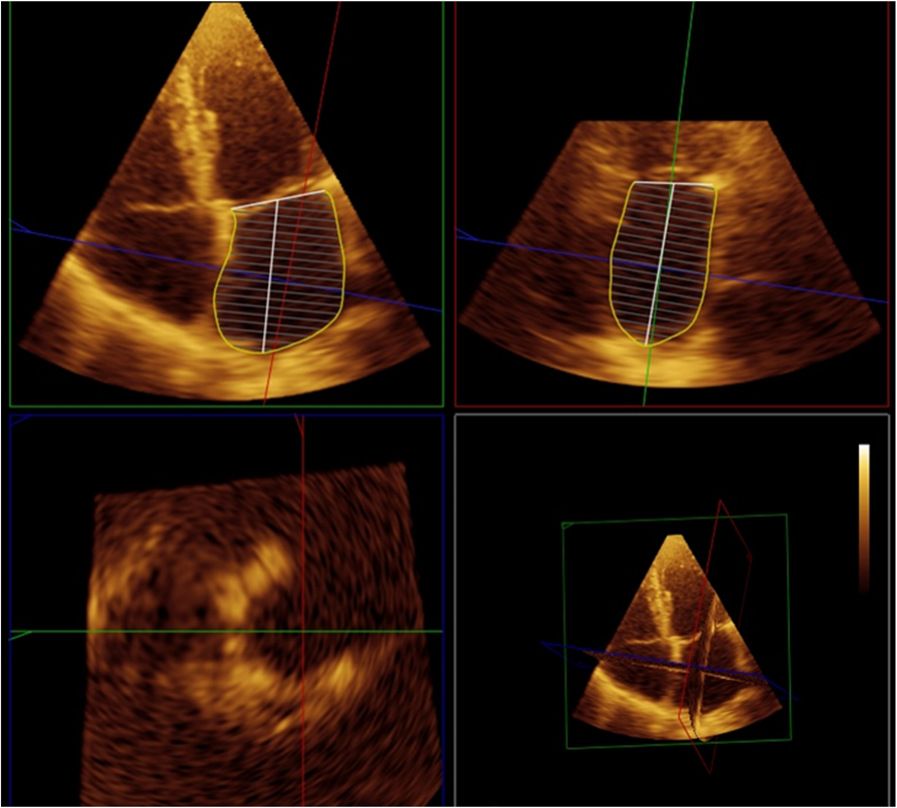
Left atrial volume measurement by 3D transthoracic echocardiography. Left atrial volumes (LAV) were measured by freehand 3D–transthoracic echocardiography using a dedicated software for left ventricular analysis (QLAB, 8.1, Philips Deutschland GmbH, Hamburg, Germany). Care was taken to manually adjust the measurement planes appropriately and exclude the pulmonary vein ostia and the left atrial appendage from the LAV at baseline, as well as at follow up controls after the procedure
